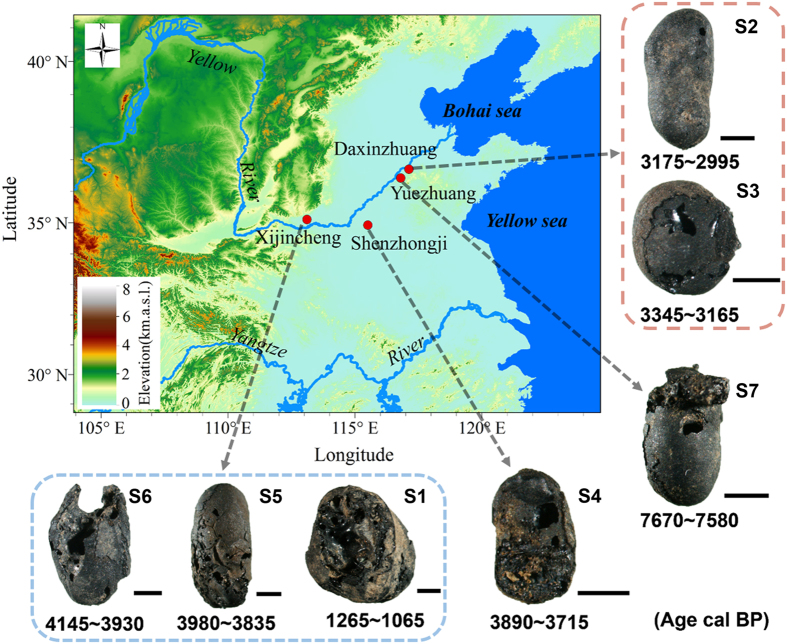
Location of sites from which CT-scanned archaeological soybeans were recovered. The numbers below each soybean image indicate AMS dates (cal B.P., 2- sigma range). Scale bar: 1 mm. The map was created with ArcGIS v.10.1 (ESRI, http://www.esri.com/software/arcgis) and annotated by the authors.
